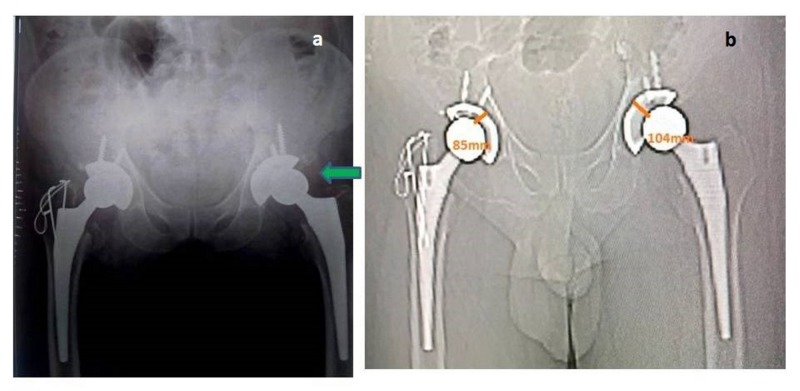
Imaging study. a) X-ray pelvis of the bilateral hip showing a concentric reduction on the right side
